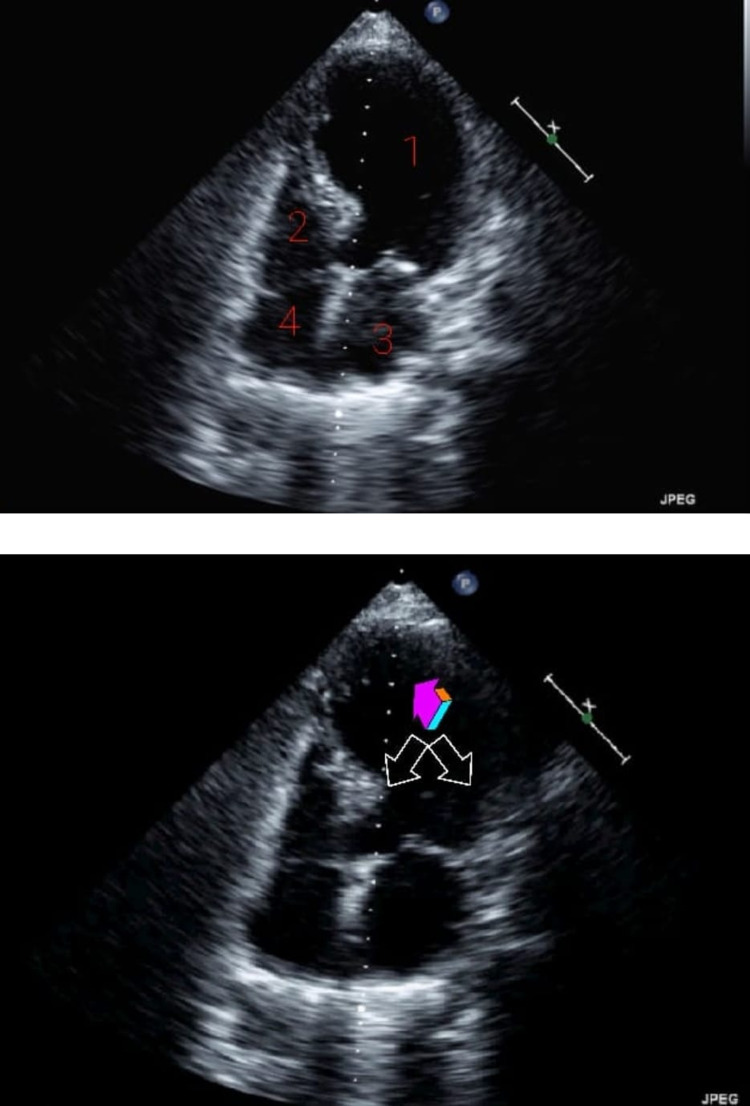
Echocardiogram (TTE) with four-chamber view of the heart. Top: 1. left ventricle (LV), 2. right ventricle (RV), 3. left atrium (LA), 4. right atrium (RA). Bottom: Single-colored arrow mid-apical segment ballooning. Double-arrows proximal/basal septum contracting inward.
